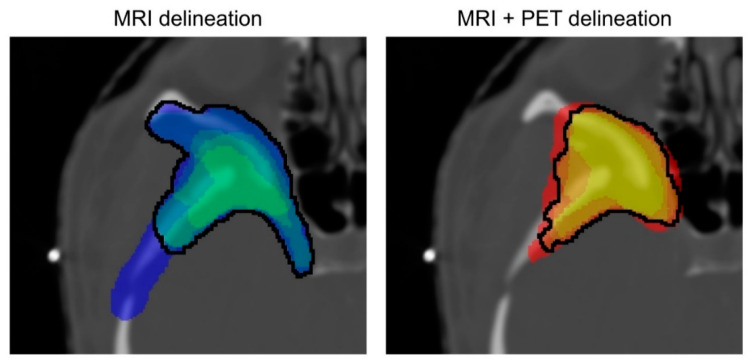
Example of the different possible consensus definitions given for the MRI and MRI + PET delineations. The color coding differentiates the amount of consensus between the physicians. The black curves outline the contours, which were created by using the STAPLE algorithm. For the MRI + PET delineation, the differences between the consensus delineations are smaller compared to the delineations based solely on the MR images.
